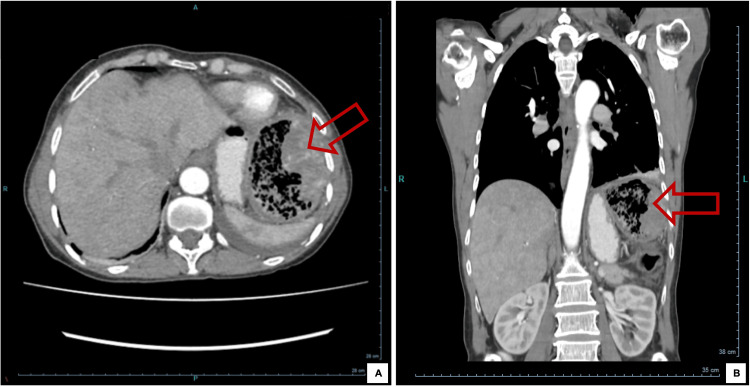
Abdominal CT angiography, axial section (A) and coronal section (B) Figure on mediastinal window showing necrotic mass with a gaseous component and vascularized in the upper left quadrant of the abdomen, subdiaphragmatic, with 9 cm of longest axis, of probable colic origin.
